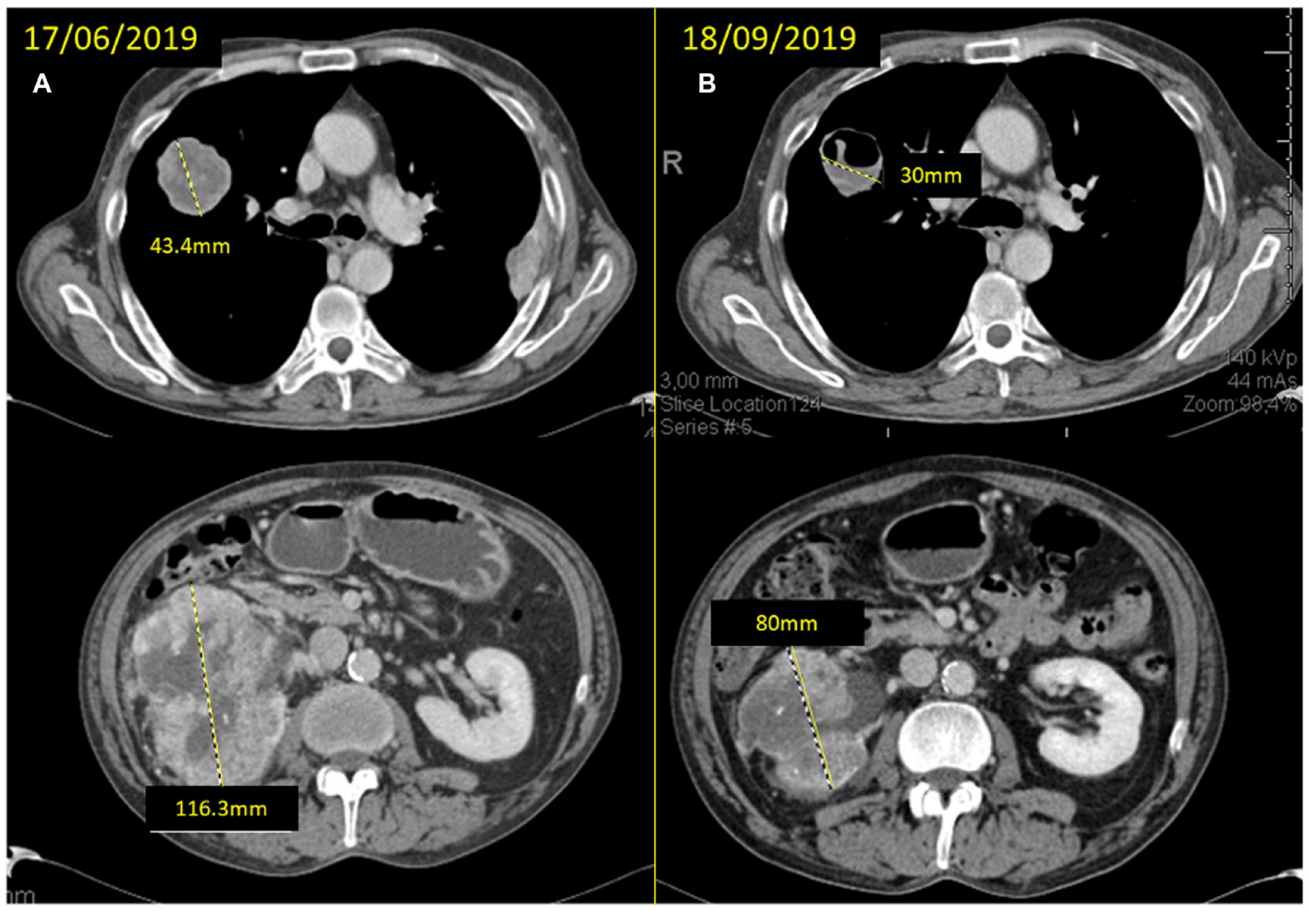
(A) baseline CT scan and (B) response after 12 weeks of neoadjuvant treatment with cabozantinib with significant reduction of the mass in the superior right lobe (SRL) from 43.4 to 30 mm and of the right kidney mass from 116.3 to 80 mm.
